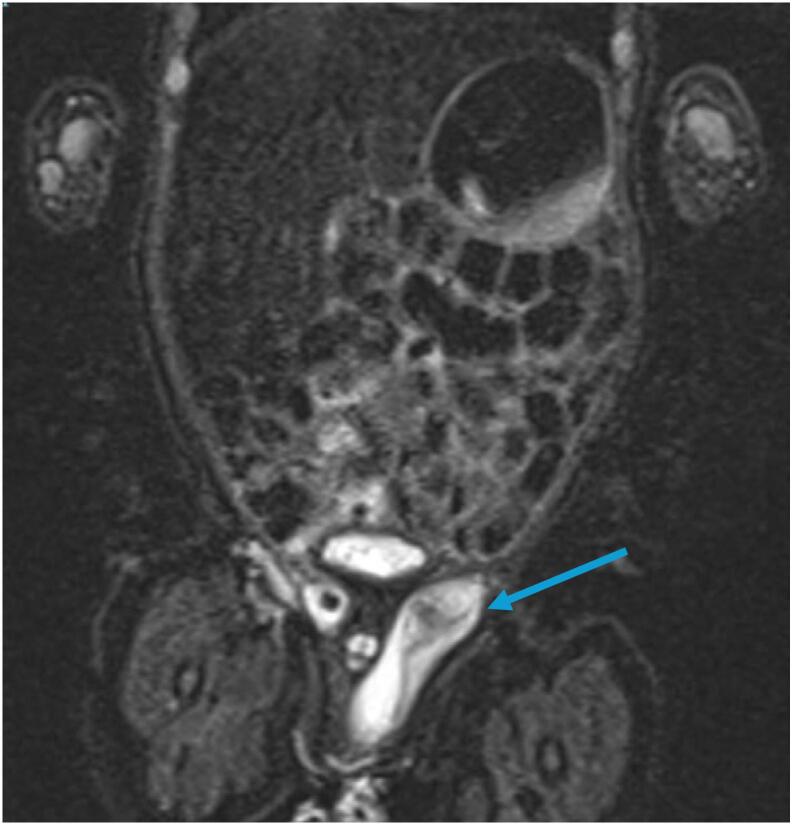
(Right) A coronal section of the abdominopelvic MRI scan demonstrating a left indirect inguinal hernia (blue arrow) initially reported as a left-sided scrotal sac containing small non-dilated bowel loops proximally and possible undescended left testis. Indication for the scan was to investigate for possible VSC in a 1-week-old phenotypical male with TGA. Clinical history included a normal penis, palpable gonad in the left groin and empty scrotum on examination combined with a uterine structure seen on an early postnatal US scan. Of note, no female genitalia (uterus or ovaries) were identified on this MRI. (For interpretation of the references to colour in this figure legend, the reader is referred to the web version of this article.)
